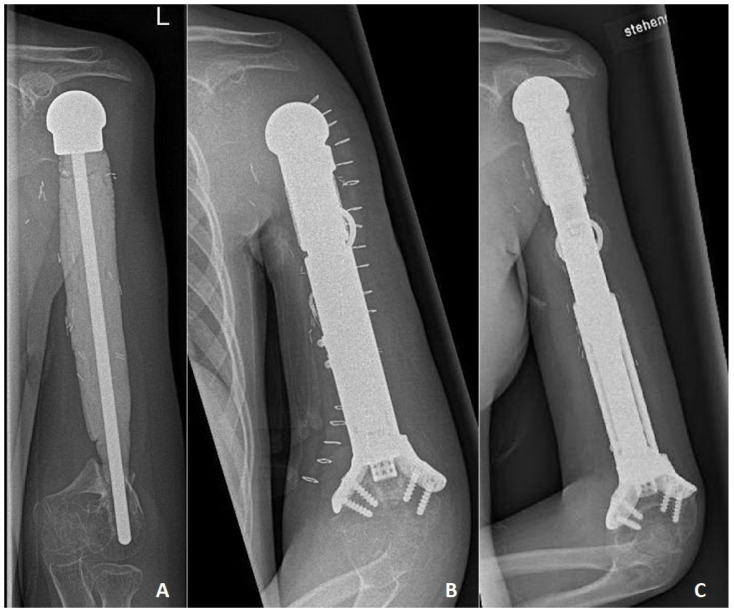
Due to osteosarcoma located in the left proximal humerus (case no 2), a cement spacer was applied after resection, and the patient presented with increased luxation of the distal part of the spacer after 62 months. (A) preoperatively a.p. left humerus X-ray. (B) X-ray a.p. left humerus, revision and re-construction with a customized expandable prosthesis with distal hollow stem. Locking screws and external implant faps that increase the contact surface and stability. (C) X-ray a.p. left humerus at 50-month follow-up (prosthesis was extended by 5 cm).
